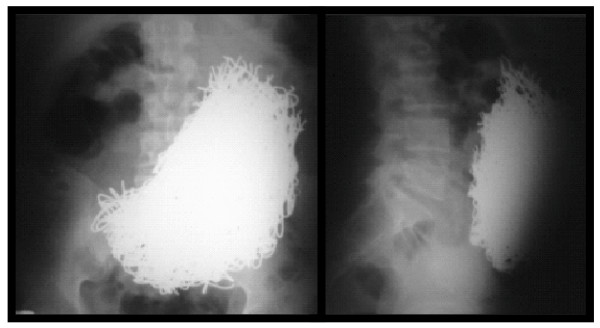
Plain radiographs (anteroposterior and lateral) of the abdomen showing multiple objects of metal density contained within the stomach.
